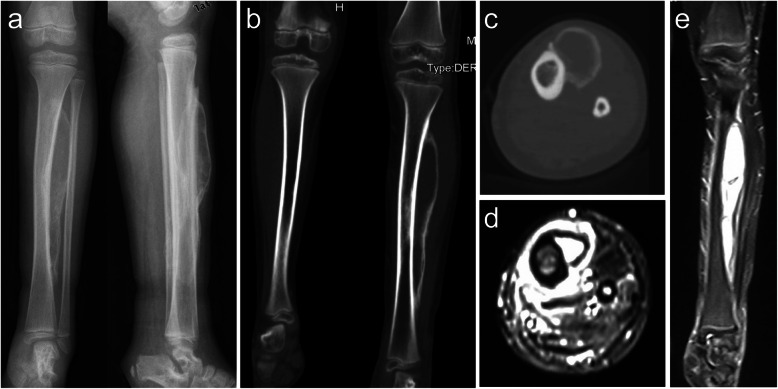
Radiographs and computed tomography images of the tibiofibular (obtained 7 weeks after the initial radiograph) reveal a parosteal high-density lesion with well-defined borders along the long axis of the left tibia (a, b, c). T2-weighted magnetic resonance imaging scans of the lower legs show heterogeneous high-signal intensity in the anterolateral aspect of the tibia, with diffused dilated vessels in the left shank ( d, e)
